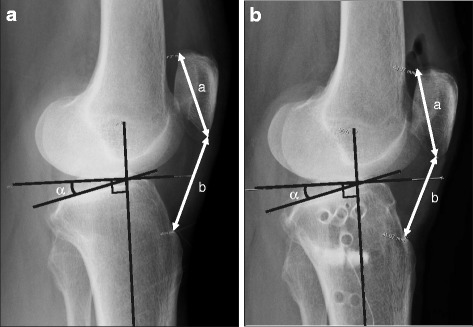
Tibial slope angle and Insall-Salvati ratio. These indicators were measured on the lateral radiograph of the knee joint. a Before surgery. b After nail extraction at 1 year after opening wedge high tibial osteotomy. *α* Tibial slope angle, *b/a* Insall-Salvati ratio
